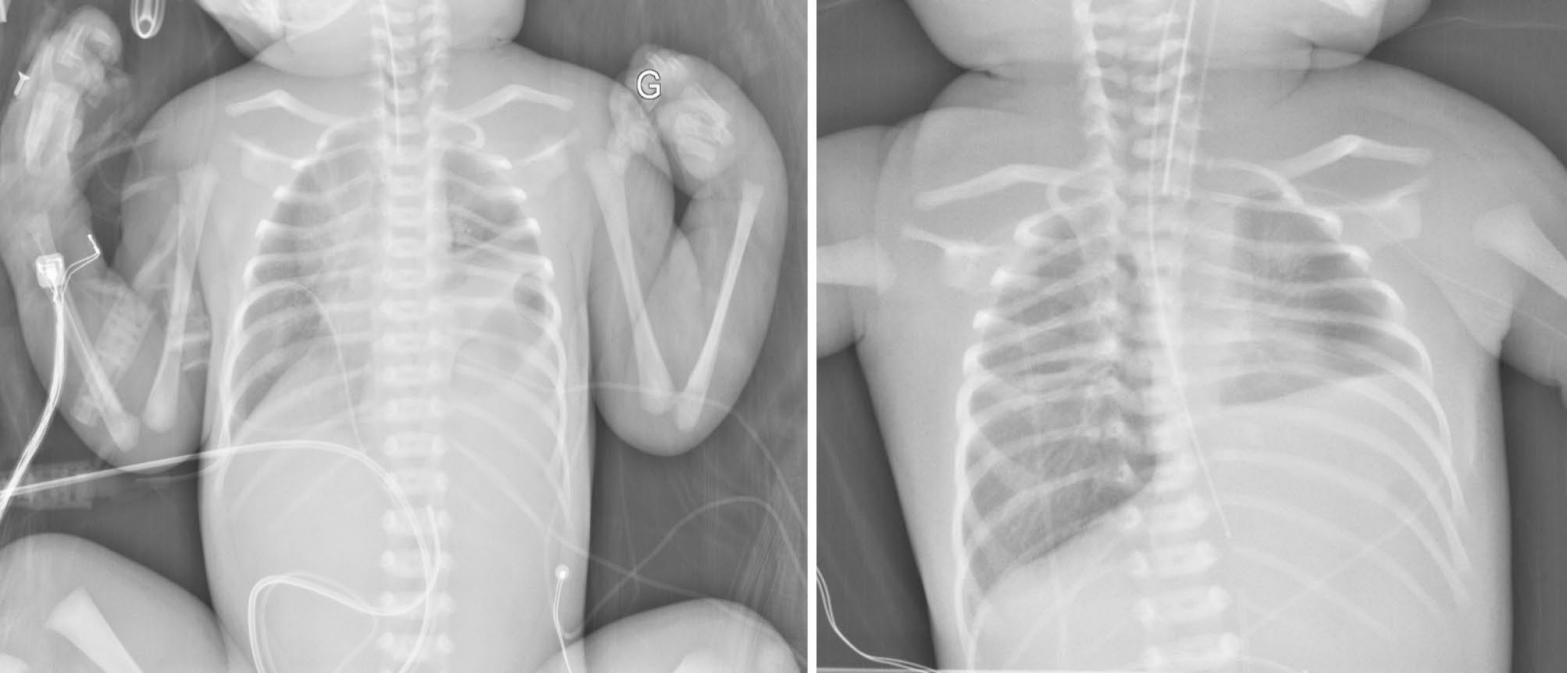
Chest X-ray at day 1 (left panel) and day 5 (right panel) of life. Chest radiography shows the bowel loops occupying part of the left hemithorax the 1st post-natal day, and a relatively preserved lung volume, especially on the right side, the 5th post-natal day
